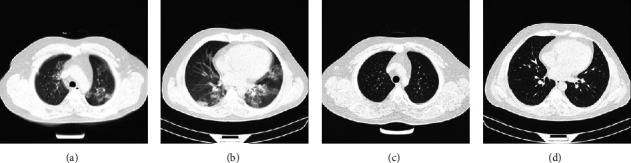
A 32-year-old man with COVID-19 pneumonia treated with oseltamivir, steroid pulse, and hydroxychloroquine. The on admission chest CT scan shows patchy ground-glass opacities (a, b) with a 10/25 severity score. The follow-up CT scan about eight weeks after hospital discharge shows no residual opacity. (c, d).
